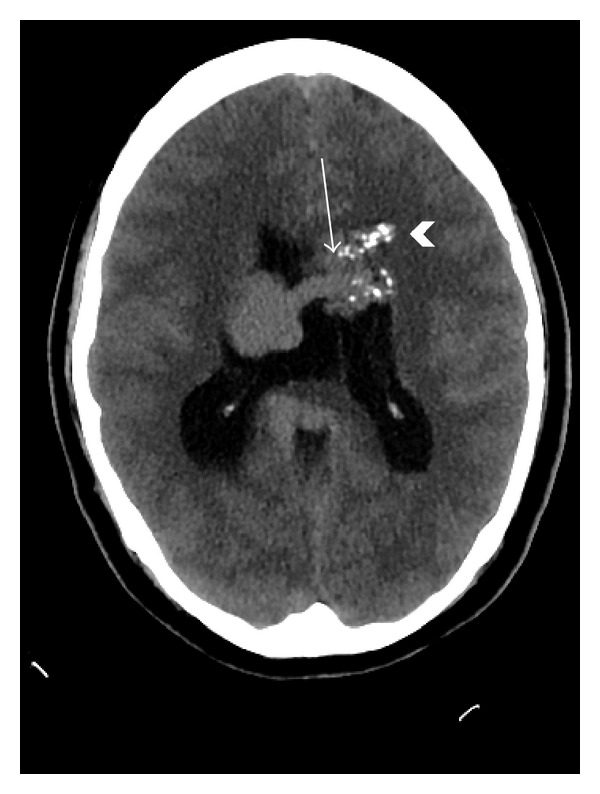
Axial noncontrast enhanced CT of the brain demonstrating a serpiginous intraventricular structure (arrow) with calcifications (arrowhead).
